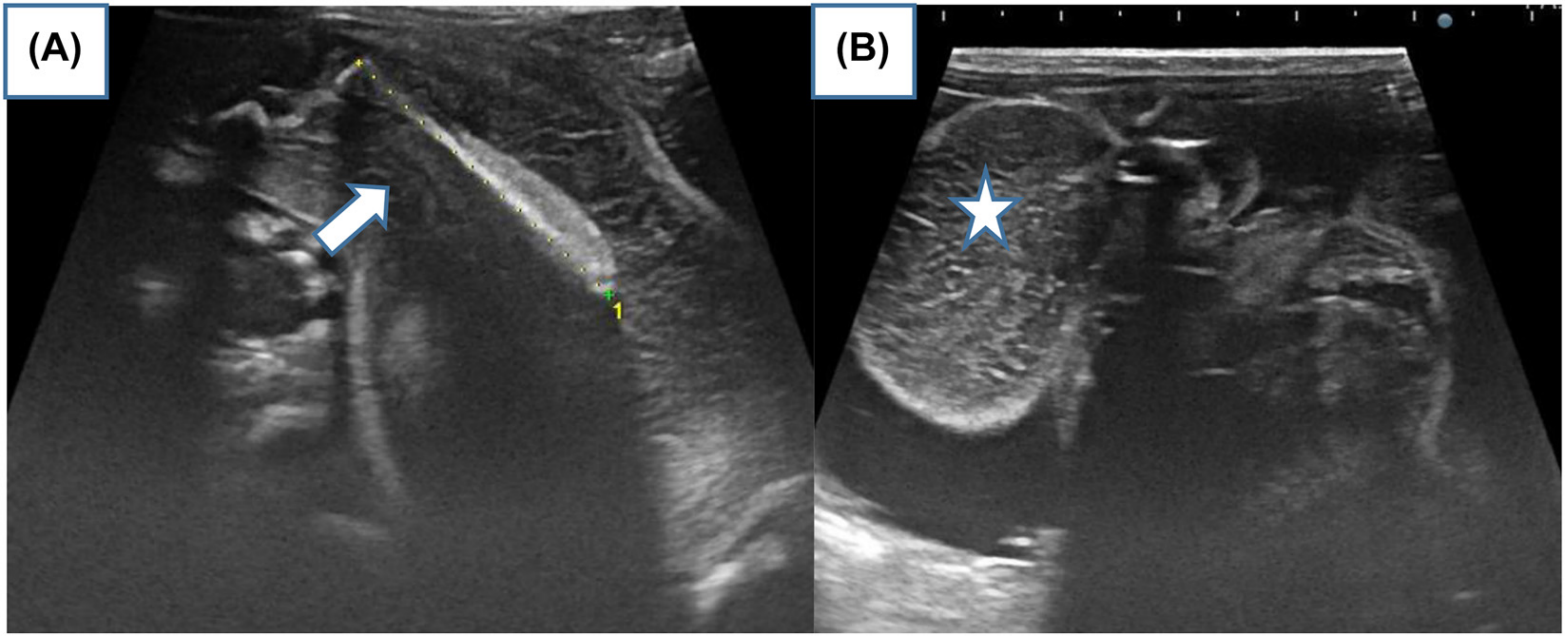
(A, B): Postnatal ultrasound of the newborn shows show heterogeneously echogenic mass with linear echogenic structure resembling bone (arrow) and intestines (star).
